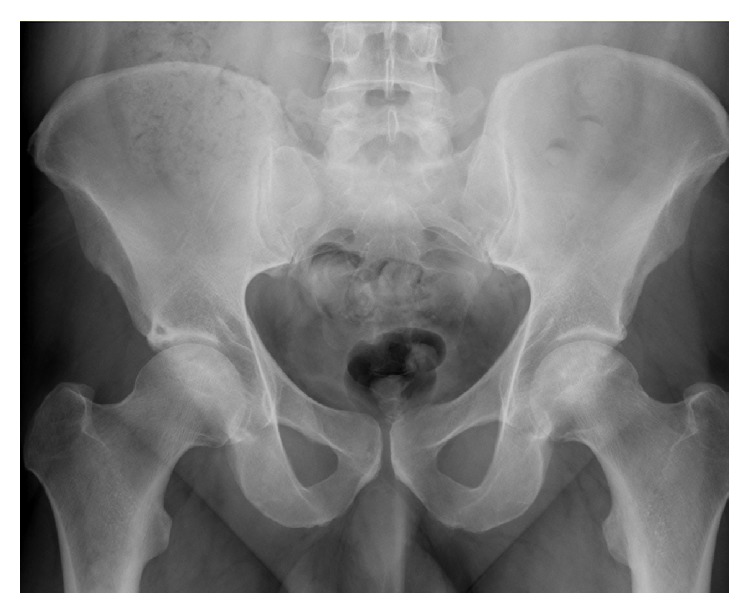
Anterior-posterior hip X-rays in patient number 3 at preanticoagulation study entry: both hips are Ficat stage II.
